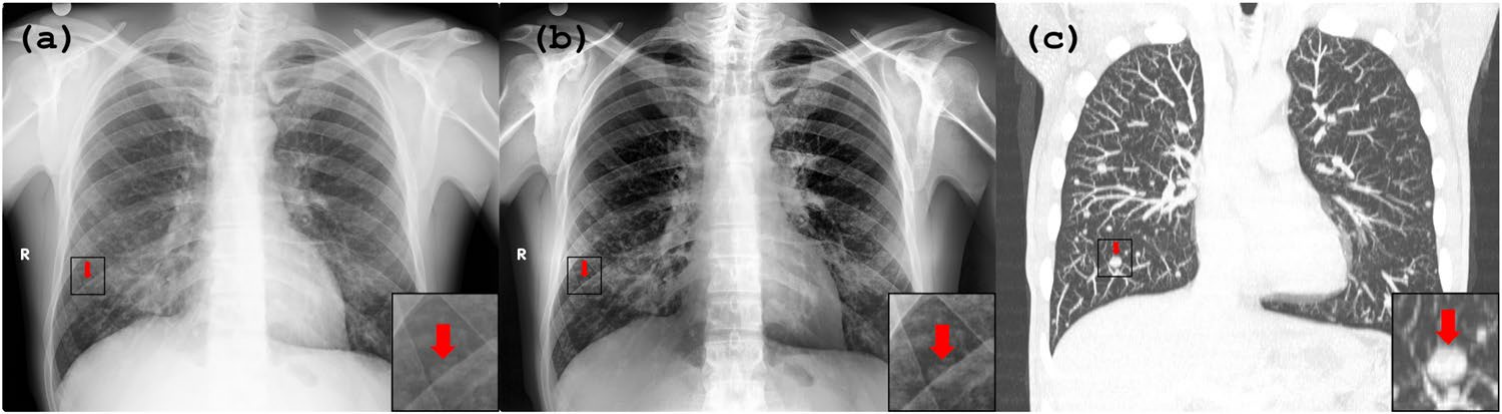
The patient in question has multiple lung metastases resulting from malignant paraganglioma. The image set comprises (a) the original anteroposterior CXR, (b) the image obtained after applying PACE2.0, and (c) the corresponding CT image. The initial chest radiograph displays haziness in both lungs, with multiple small nodules that are poorly defined (red arrow), resulting in low diagnostic confidence. In contrast, the nodules are more apparent in (b), the PACE2.0-processed image, where there is a noticeable improvement in the edge definition of one nodule (red arrow), which can be seen more clearly in the insets. The apparent difference of position of nodule between the X-ray image (a and b) and CT scan (c) is due to the different position of the patient during acquisition of images (supine on CT and in standing position on x-ray) and consequent differences in inspiration depth. The reconstructed coronal chest CT image (c) confirms the presence of multiple nodular (red nodule) metastases in both lungs.
